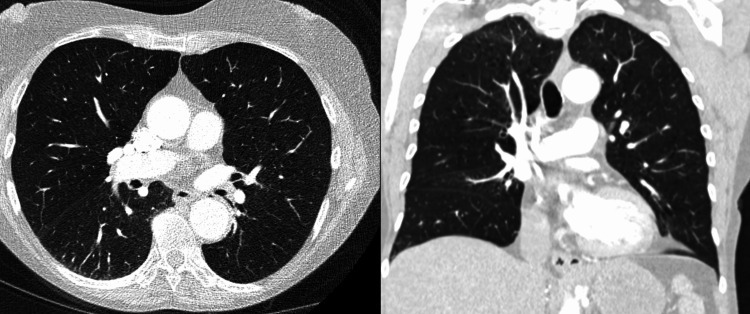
Interim CT scan of the chest The interim chest CT scan shows no evidence of pulmonary edema or pleural effusions, indicating that pulmonary edema developed later in the clinical course. Left: axial view; right: coronal view.
